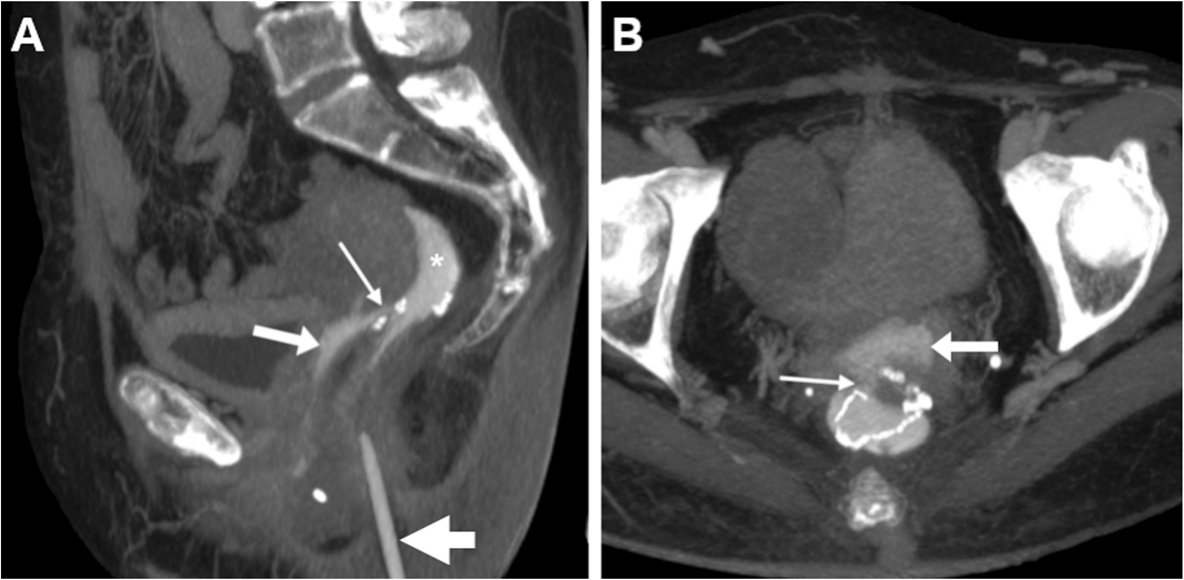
CT with retrograde contrast medium (CM) enema in VF following low anterior resection (LAR) for rectal carcinoma: maximum-intensity projection (MIP) CT reconstructions (a, b) show contrast medium flowing from the diverted stump into the vagina (arrows) through the patent VF (thin arrows). Note rectal catheter (thick arrow in a)
